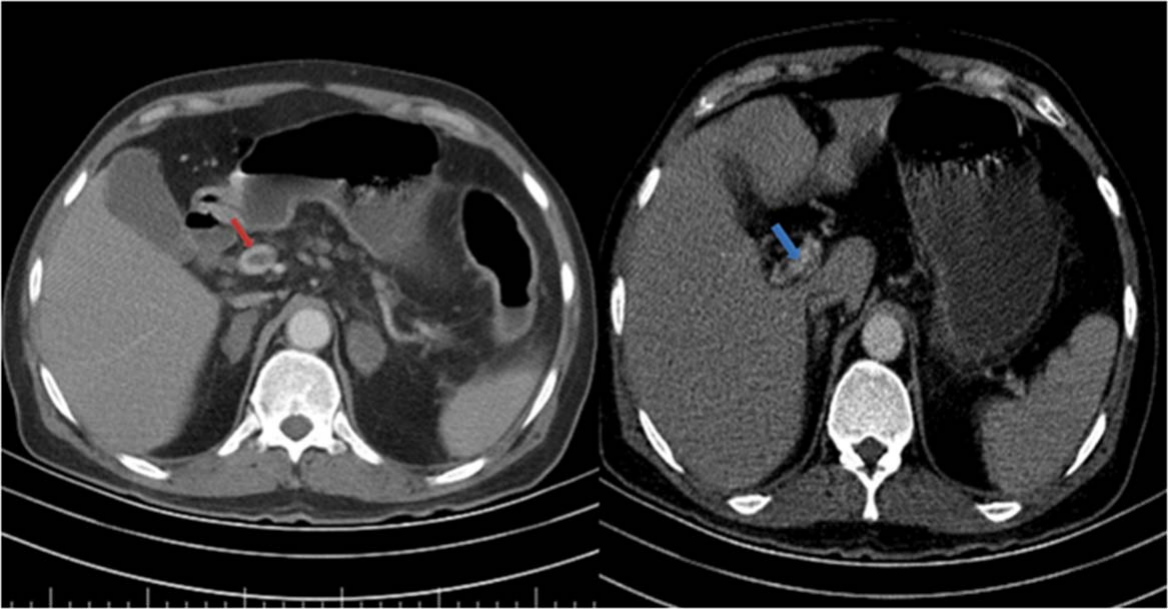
Axial contrast-enhanced abdominal computed tomography examination shows filling defects in the lumen of the portal vein, suggesting portal vein thrombosis. Red arrow shows arterial phase and blue arrow shows venous phase
